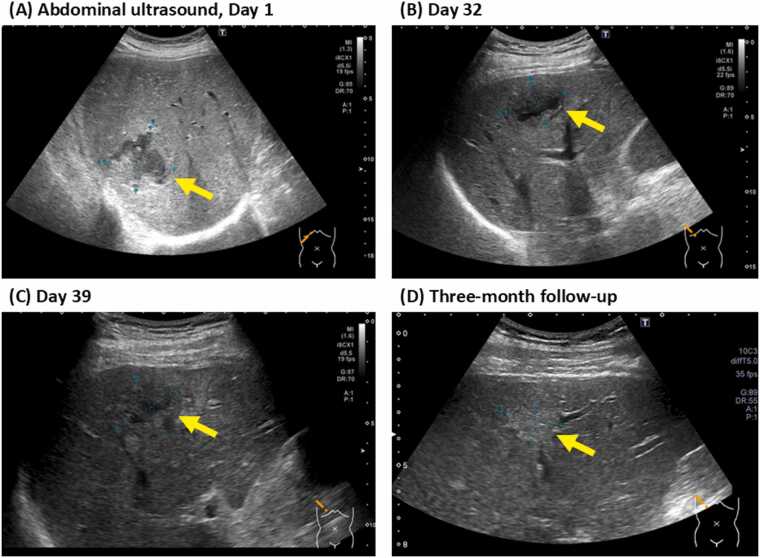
Serial abdominal ultrasound findings of the liver abscesses during antibiotic treatment, (a) On day 1, the largest abscess measured 50.4 × 38.8 mm. (b) On day 32, the abscess remained at 40.6 × 27.2 mm. (c) (C) On day 39, the abscess had decreased in size to 33.7 × 29.7 mm, following continued intravenous antibiotic therapy. (d) (D) At the three-month follow-up, abscess size reduction to 21.6 × 12.0 mm with residual scarring was confirmed.
